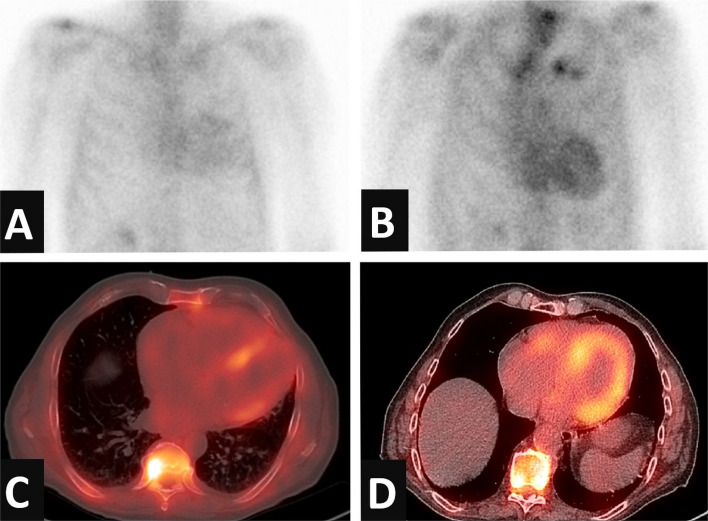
Scintigraphy with technetium-99 m pyrophosphate. A, B Planar scintigraphy images. C, D Single-photon emission computed tomography/computed tomography fusion images. A, C Images of a patient with Perugini grade 2 uptake. B, D Images of a patient with Perugini grade 3 uptake
